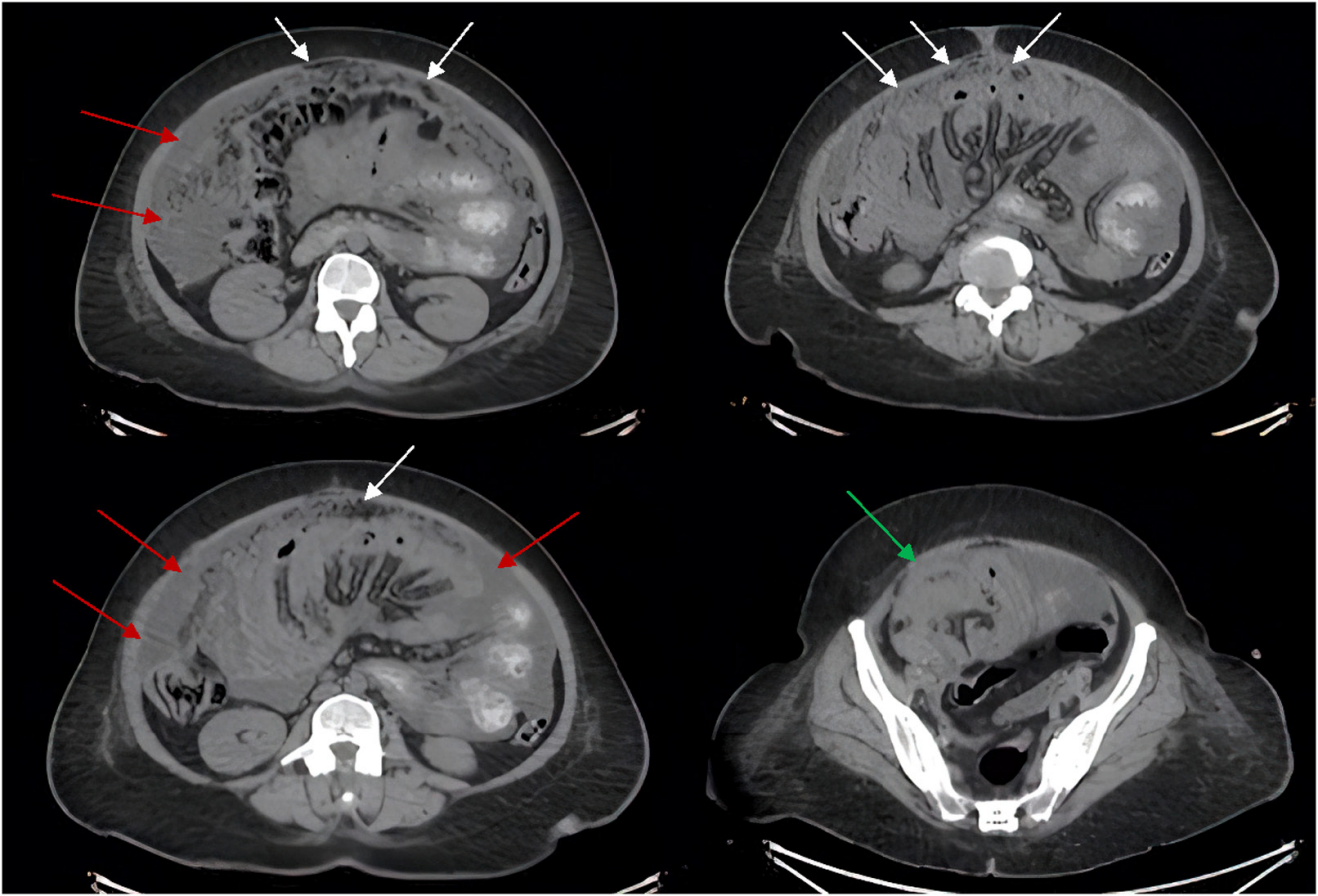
Cross‐sectional (transverse) abdominal and pelvic CT with contrast. It shows diffuse omental caking (White arrows) with thick enhancing peritoneal reflections (Red arrows) and soft tissue thickening of the right adnexa with a poorly defined ovary (Green arrow).
